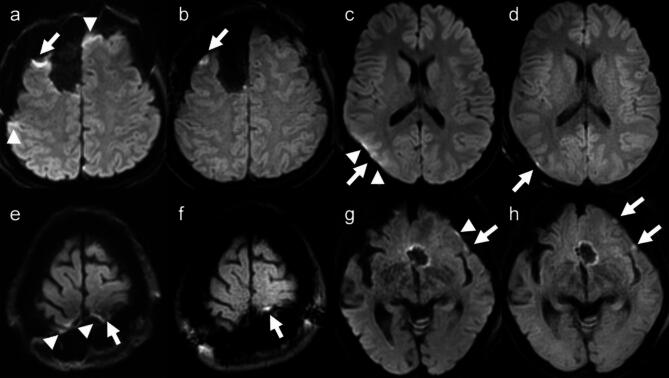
Representative images obtained in patients who underwent surgery for brain tumor show postoperative changes related to acute cerebral infarction or postoperative contusion on postoperative day 1. It is difficult to determine whether the postoperative changes are due to acute cerebral infarction or to postoperative contusion (white arrows) and susceptibility artifact (arrowheads) in SS-EPI DWI (a, c, e, and g). Postoperative changes are clearly differentiated as acute cerebral infarction or postoperative contusion on TGSE-BLADE DWI (b, d, f, and h).
